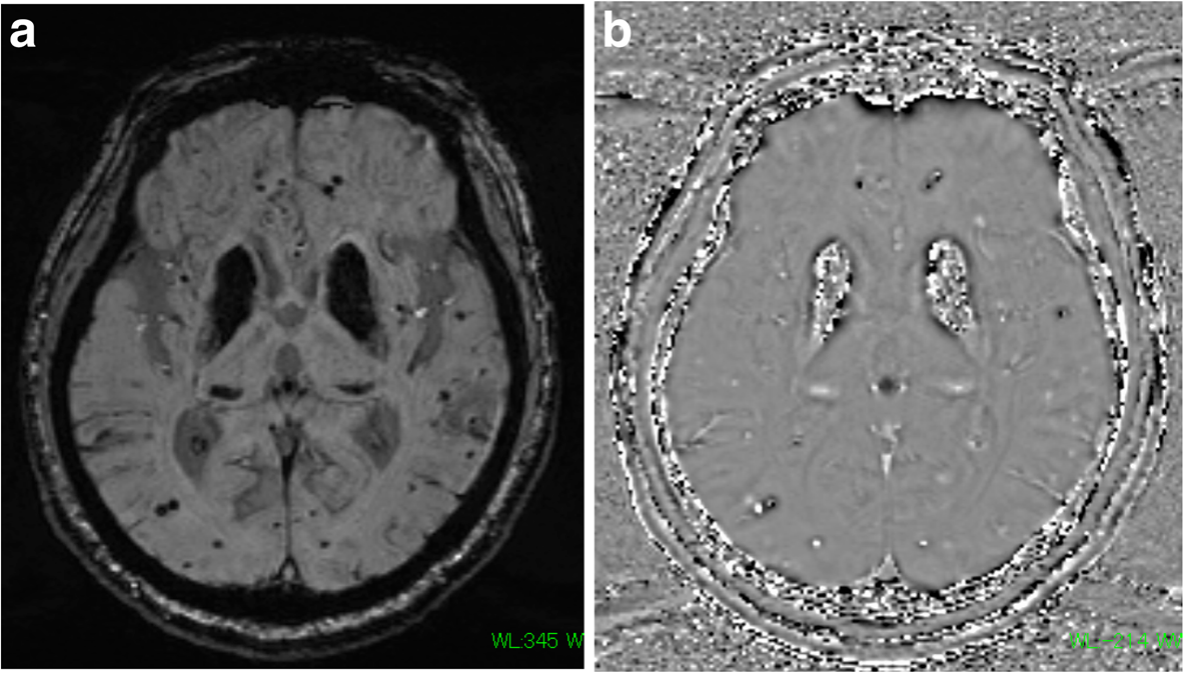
Brain magnetic resonance imaging (MRI). Minimal intensity projection (a) and phase images (b) on SWI showing multiple hypointense spots in the cerebral sulcus and hypointense lesions coinciding with high-density lesions on CT
